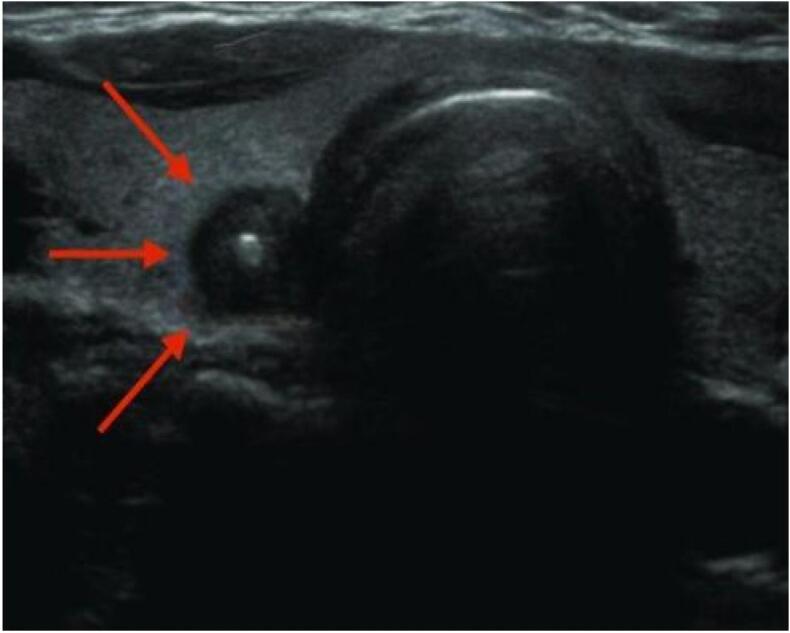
Neck ultrasound showing a 1 cm thyroid nodule (indicated by arrows) with no other abnormalities detected.
